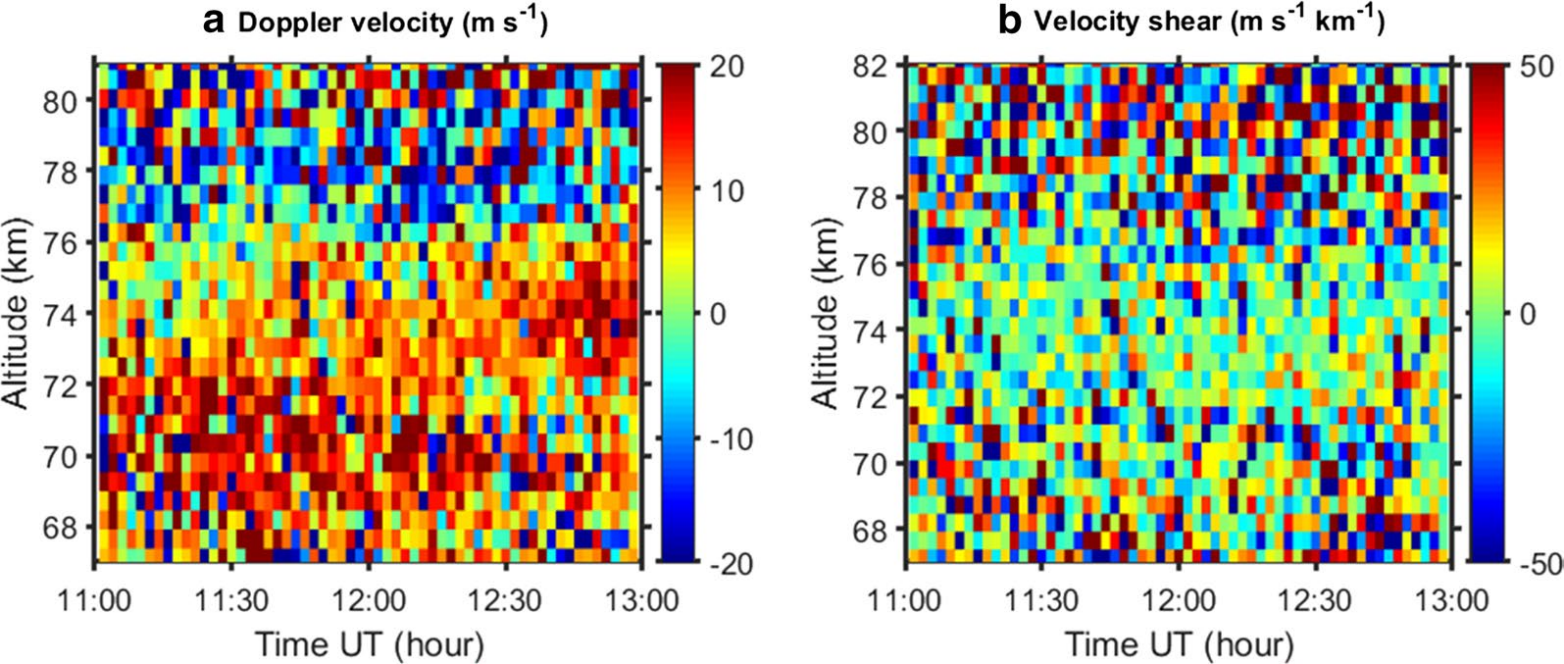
Time–altitude plots of Doppler velocity (a) and velocity shear (b) for the Kiruna receiver
